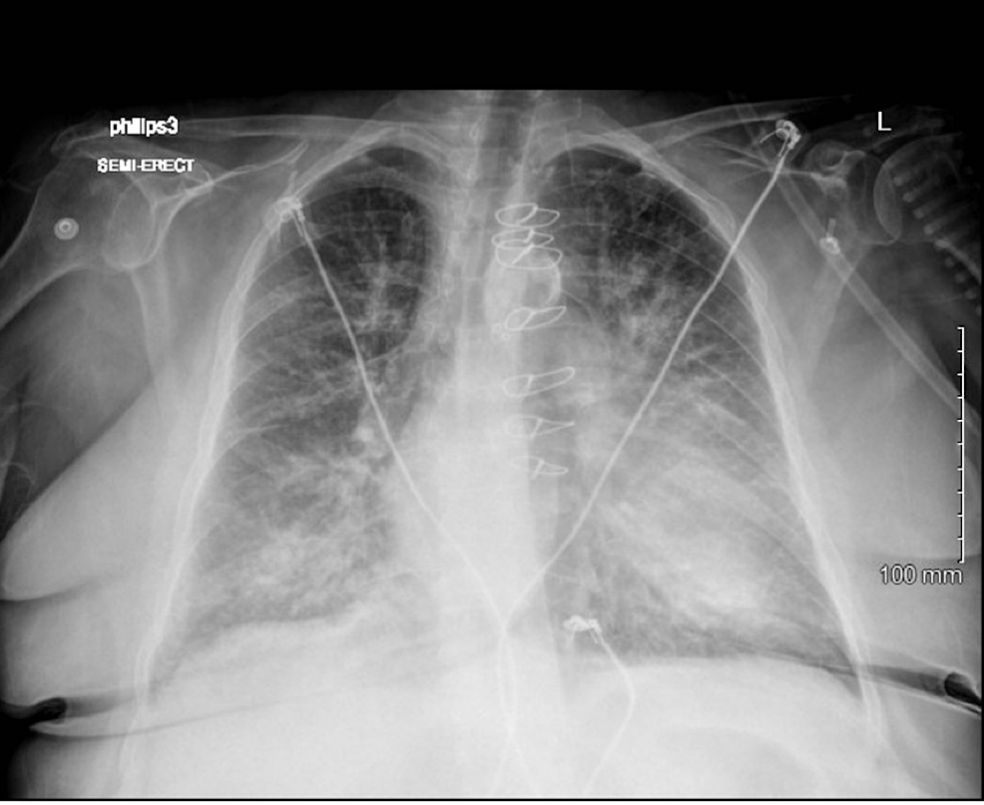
Chest X-ray after cardiac catheterization
